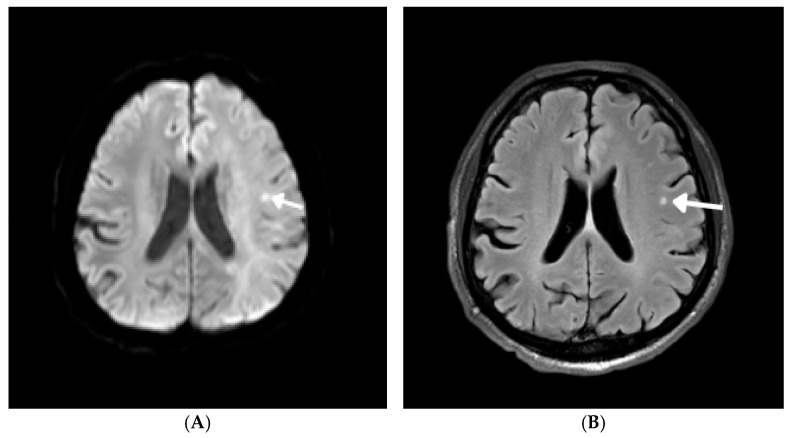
Head magnetic resonance imaging with DWI sequence (axial) (A) and FLAIR sequence (axial) (B) with abnormal hyperintense signal in the left frontal region (marked by arrow).
